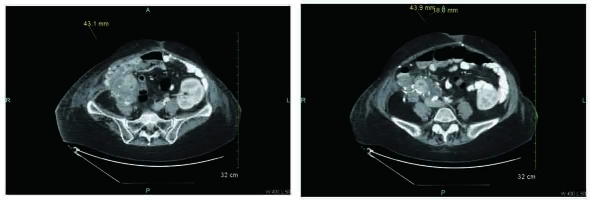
CT scan of abdomen reveals peripancreatic fluid collections with involvement of the cecal pole, findings suggestive for pancreatitis around the transplant. The pancreas transplant in the right lower quadrant is surrounded by inflammation and two fluid density areas. The inflammatory changes extend into the cecal pole area and involve this bowel loop. The inflammatory changes also extend towards the bowel, close to the urinary bladder. There is no fatty plane between the urinary bladder and the pancreas transplant with inflammation. The findings were initially concerning for pancreatitis with graft rejection.
